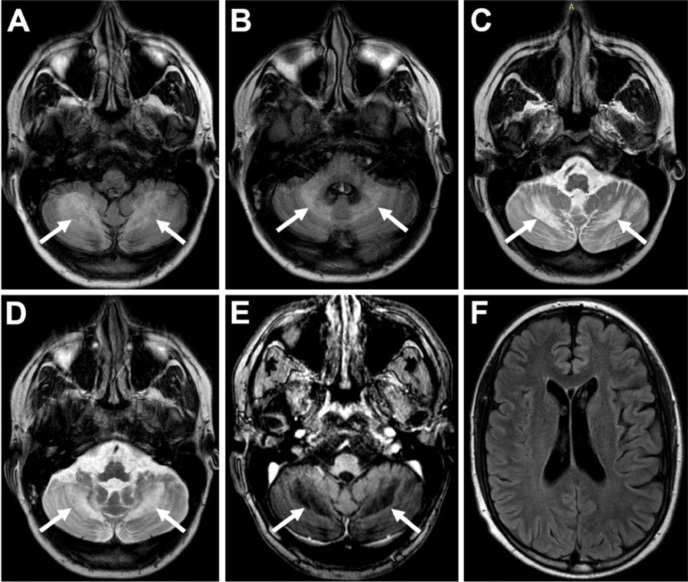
Patient with pure cerebellar ataxia related to X-linked adrenoleukodystrophy. Axial FLAIR-weighted brain MRI shows symmetrical cerebellar white matter and middle cerebellar peduncles hyperintense signal, besides pontocerebellar atrophy (A and B). Axial T2-weighted brain MRI discloses bilateral cerebellar white matter changes (C and D). Axial T1-weighted brain MRI reveals hypointense signal in cerebellar white matter (E). Axial FLAIR-weighted brain MRI shows no supratentorial abnormalities (F).
